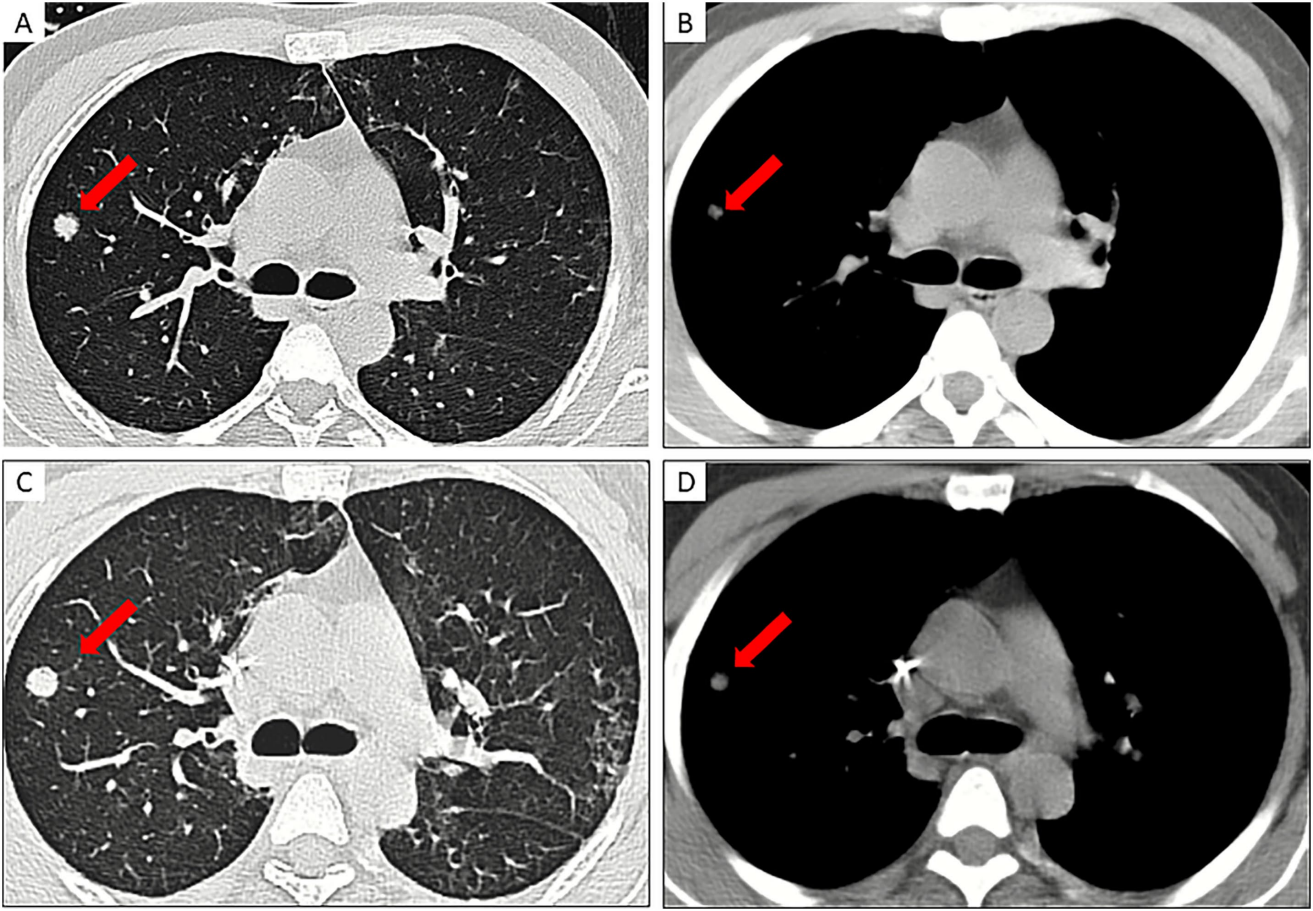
A 38-year-old male patient presented with Mpox pneumonia, initially manifesting symptoms of diarrhea and weakness. Twelve days prior to hospital admission, he engaged in unprotected homosexual intercourse. (A,B) chest CT scans with thin-section lung window (A, arrow) and mediastinal window (B, arrow) revealed a nodule in the anterior segment of the right upper lobe. (C,D) subsequent chest CT scans with thin-section lung window (C) and mediastinal window (D) indicated an increase in the size of the nodule, with greater solid component and heightened CT density of the right upper lobe nodule to 58 Hu, exhibiting more defined margins compared to the previous examination.
